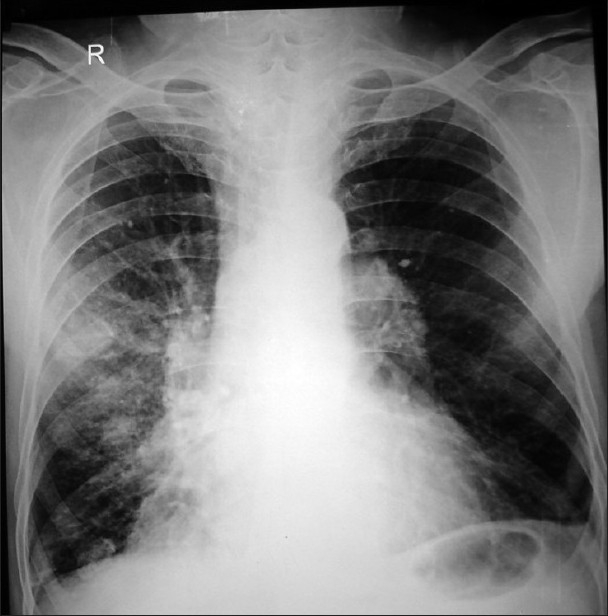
Chest radiograph showing bilateral hilar prominence
